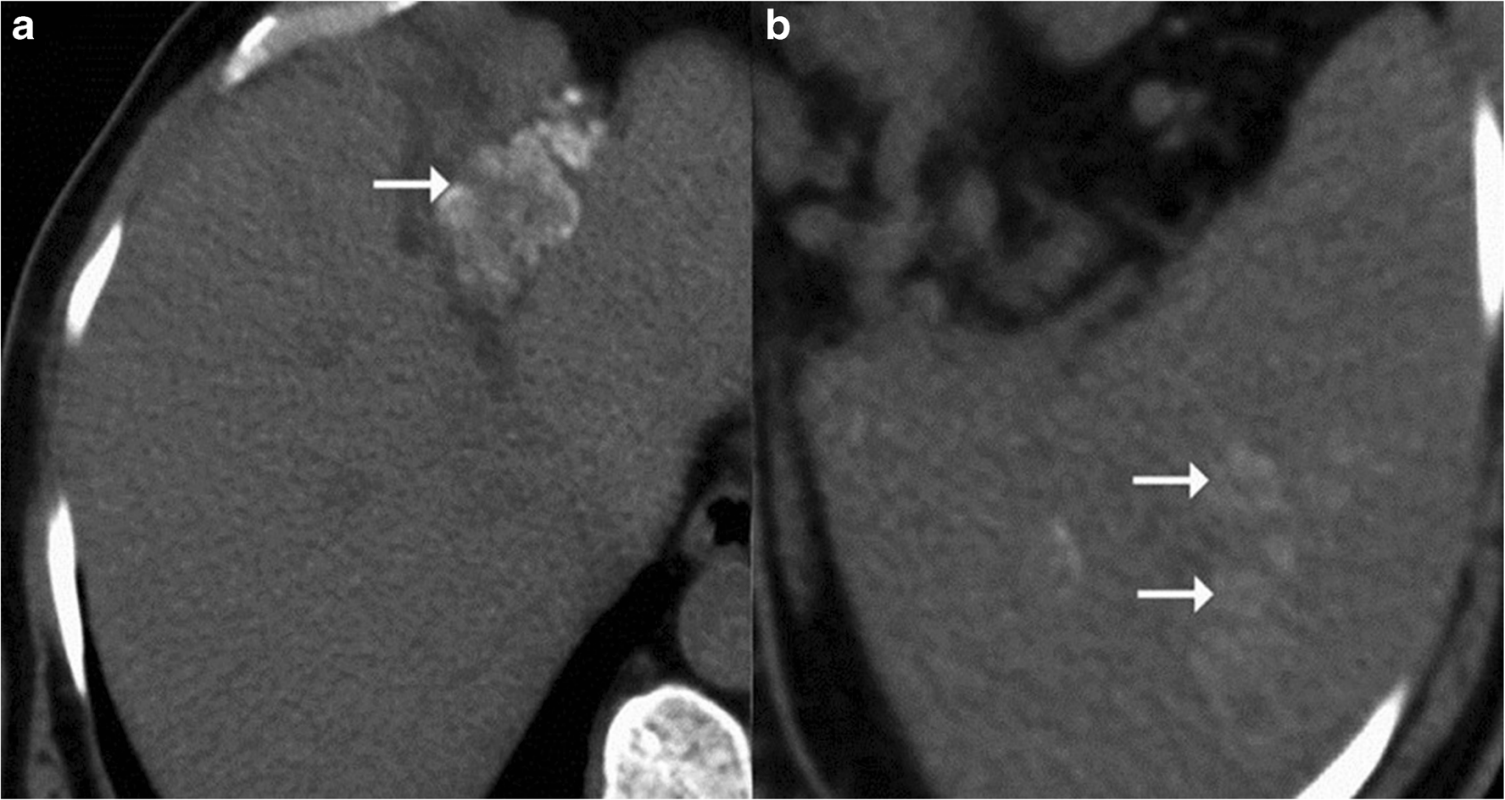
a, b Plain CT images shows lesion with dense nodular calcification in the left lobe up to the interlobar fissure with concomitant multiple calcified lesions in spleen (*arrows*)
